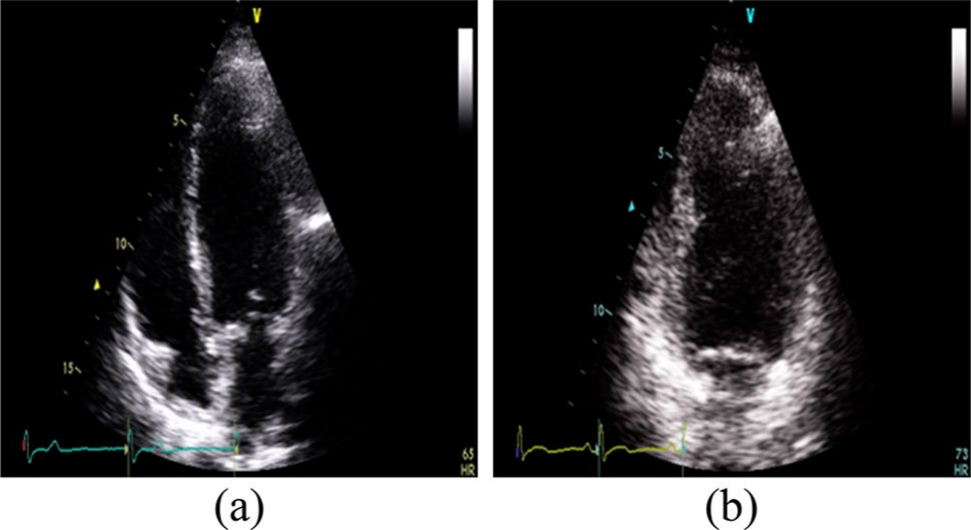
Examples of images for the echo-window classification model. (a) Apical 4-chamber. (b) Apical 2-chamber.
